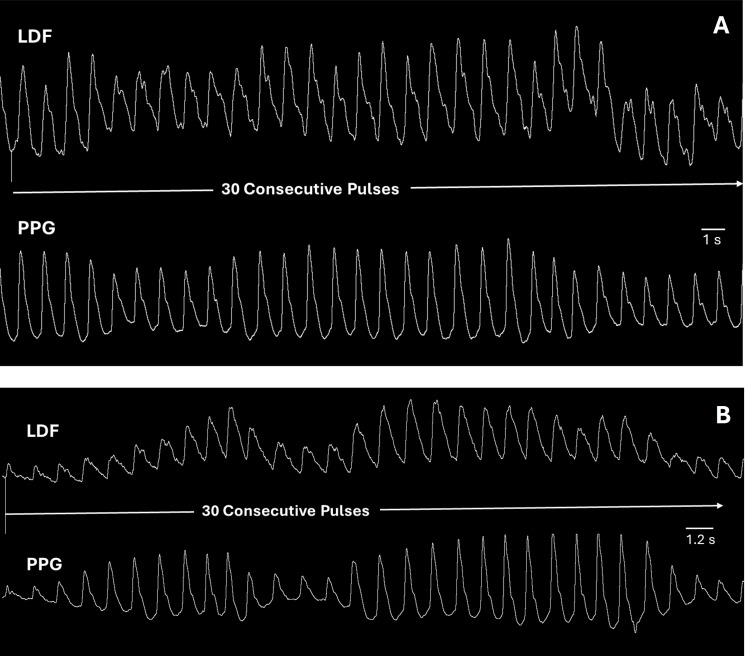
Example measurement sequences LDF is the laser Doppler flux measured on the pulp of the ring finger. PPG is the photoplethysmography signal from the index finger.  Both are shown as a function of time. In A, the 30-pulse sequence that was analyzed was captured 1496 seconds into the experiment. In B. the 30-pulse sequence that was analyzed was captured 4.5 seconds into the experiment. In both cases there is a spontaneous waxing and waning of the PPG signal with associated changes in the pattern of the LDF signal.
